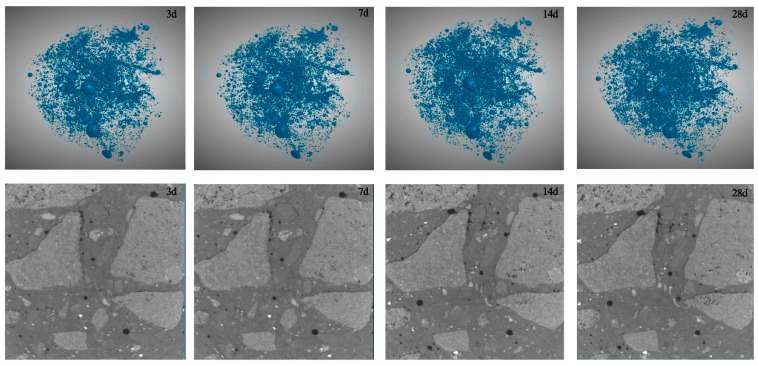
X-CT Image of SCC with 6% CAI dosage.
